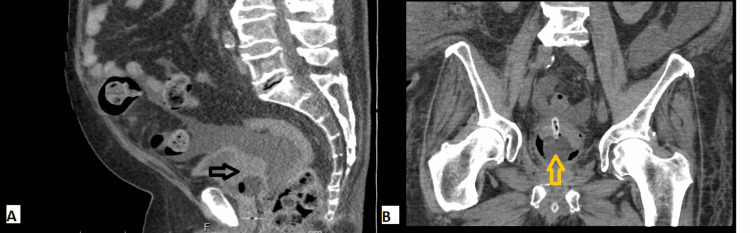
Sagittal view (A) and coronal view (B) of CT abdomen/pelvis showing density surrounding the Foley catheter balloon within the urinary bladder measuring up to 5.1 cm, as indicated by the black arrow, possibly representing blood clot and a hyperdensity within the dependent portion of the urinary bladder which may represent hemorrhage, as indicated by the yellow arrow. Increased gas is seen within the urinary bladder. Wall thickening within the urinary bladder with adjacent stranding is noted along with a moderate amount of ascites. CT: computed tomography
